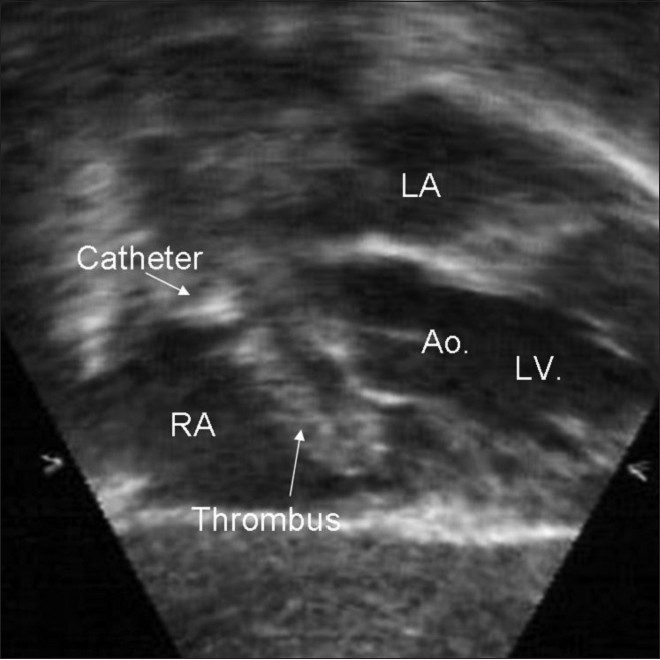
Two-dimensional echocardiography, a modified subcostal saggital view showing thrombus attached to the catheter tip in the right atrium (RA)
